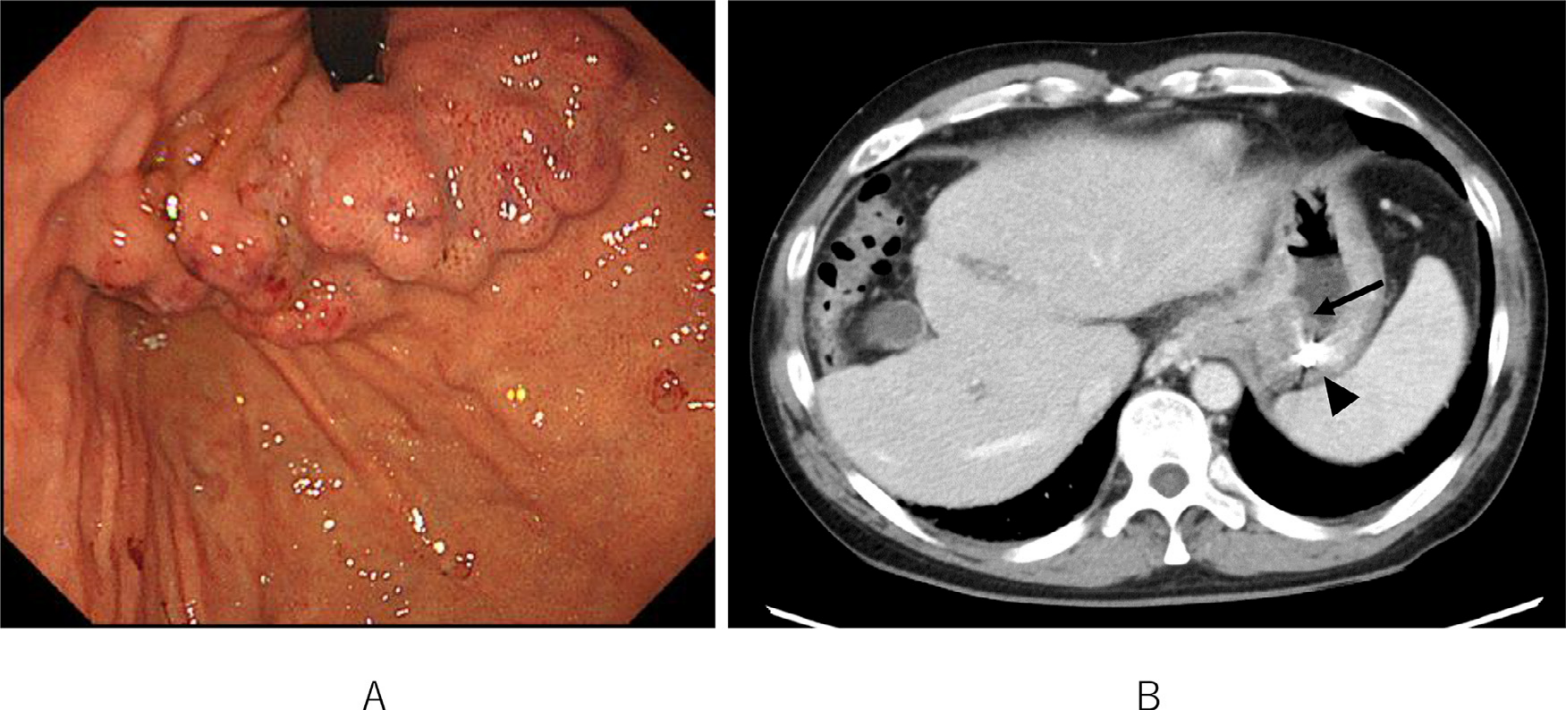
Contrast-enhanced CT and endoscopic picture after treatment. (A) Endoscopic picture 7 days after retrograde obliteration shows large gastric varices with redness which do not change the shape. (B) Contrast-enhanced CT 16 days after the procedures shows that the gastric varices are completely thrombosed (black arrow). The black arrowhead indicates the microcoils used in the TIO.
